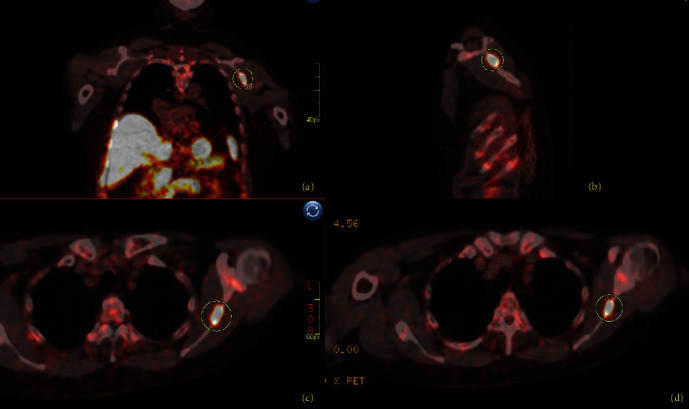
Case 1: left scapula ^68^Ga-DOTATATE-PET/CT images. Coronal (a), sagittal (b), and two axial (c and d) ^68^Ga-DOTATATE-PET/CT images with multiple foci of mild uptake noted with bilateral ribs, costovertebral junctions, and left glenoid concerning for microfractures; and area of hyperintesnse uptake within the left scapula (circle) consistent with primary PMT secreting FGF-23.
